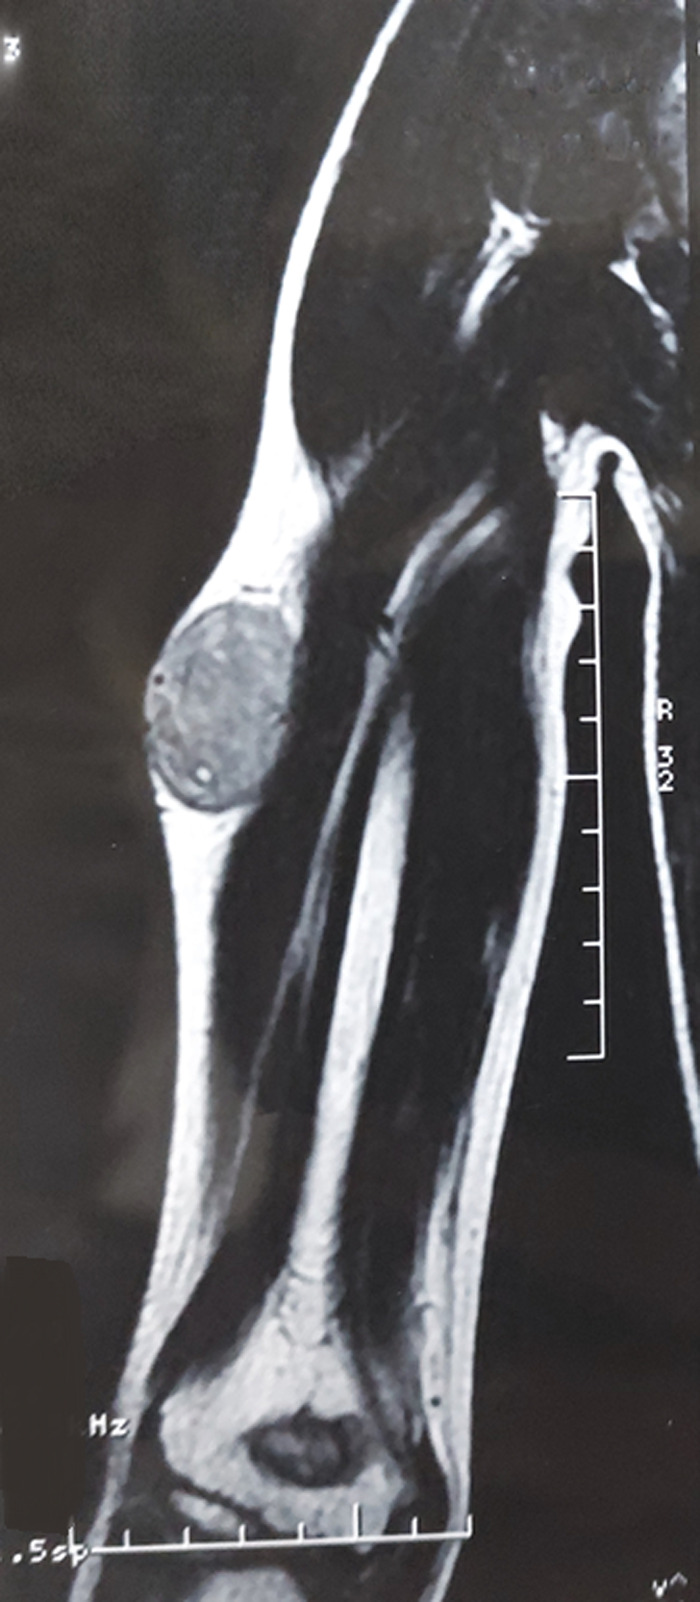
MRI of the right upper arm showing a well-limited subcutaneous mass, surrounded by a pseudo-capsule, without bone involvement
